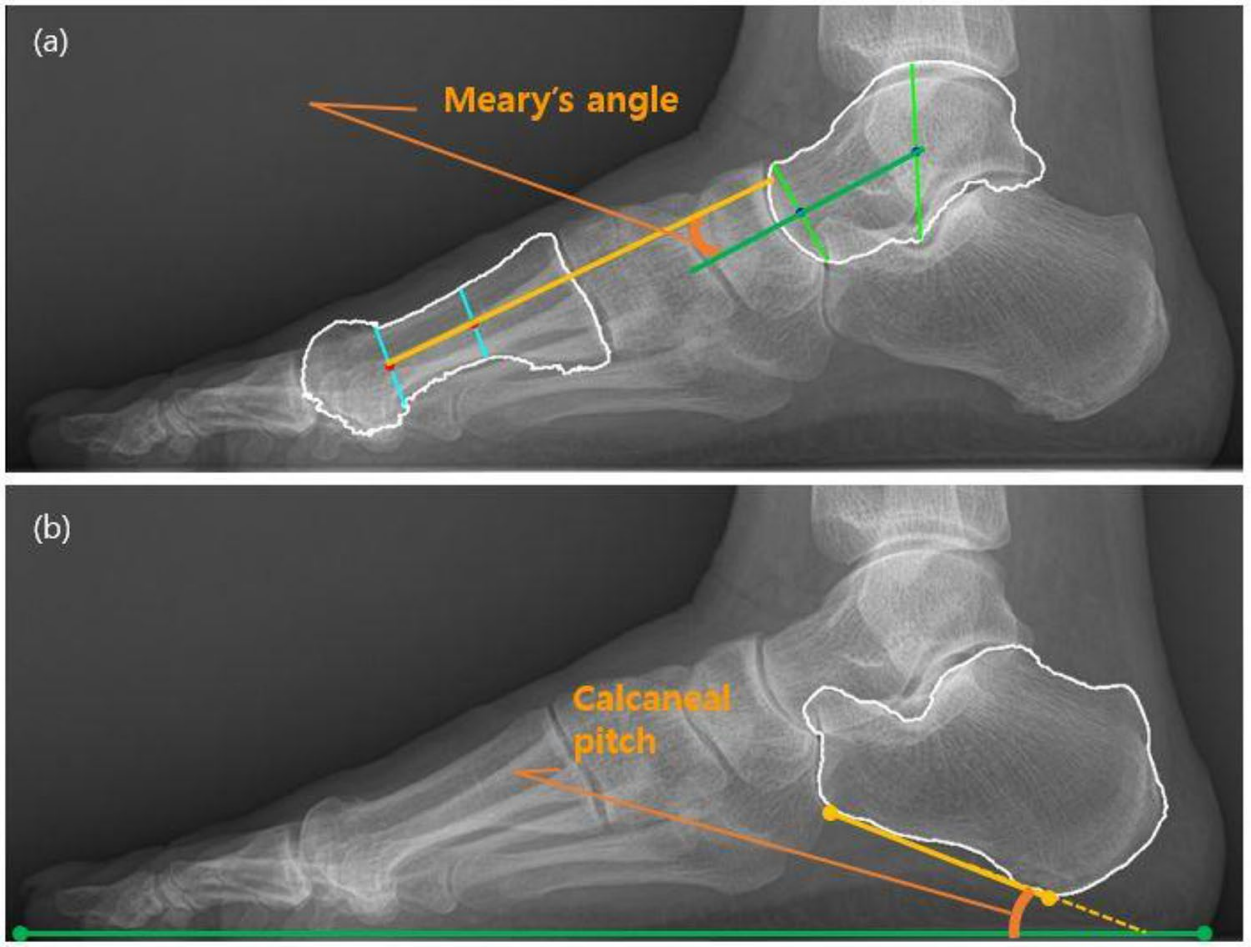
A weightbearing lateral radiograph of a normal foot of a 53 year old female, showing measurement of the Meary angle and calcaneal pitch. (a) Meary angle is the angle between the long axis of the talus and the long axis of the first metatarsus. First, the longitudinal axis of the talus is a line drawn through two mid-points. (Green line) One is a mid-point between the cephalad and caudad margins of the talar body, (Longer light green line) and the other is a mid-point between the cephalad and caudad margins of talar neck. (Shorter light green line) The metatarsus axis was determined using the following method. The long axis of the first metatarsus (Yellow line) is drawn by connecting two mid-points equidistant from the cephalad and caudad margins of the first metatarsus at the proximal and distal metaphysial diaphysial junction (blue lines). (b) Calcaneal pitch is the angle formed between the line outlining the inferior border of the calcaneus (yellow line) and the weight bearing surface (green line).
